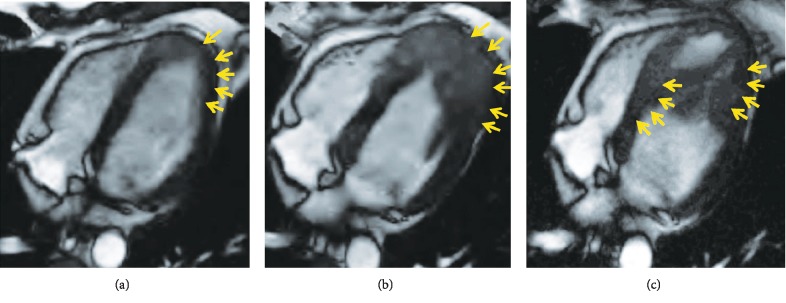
(a) Cardiac magnetic resonance imaging (MRI) demonstrates a 3.3 × 4.2 cm infiltrating lesion (arrows) within the apex of the left ventricle without early or delayed enhancement. (b) Cardiac MRI shows interval progression of the mass (arrows). (c) Cardiac MRI shows the infiltrative tumor mass involving the left ventricular myocardium with features suggesting central necrosis (arrows).
